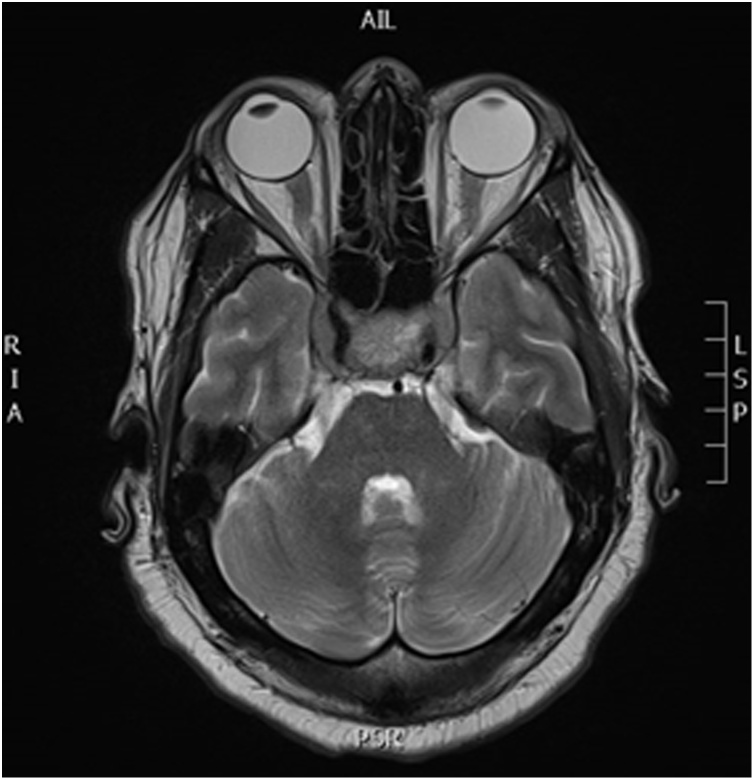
MRI brain T2 axial view showing an isotense sellar and suprasellar lesion.
